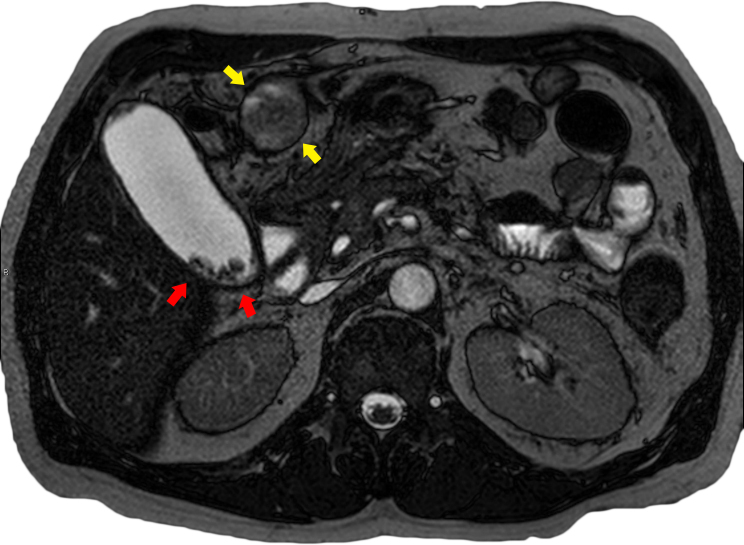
MRI *T*_2_ weighted transverse sequence again showing multiple small gallstones (red arrows). The paraumbilical vein is now significantly distended, measuring approximately 4 cm maximally (yellow arrows). Centrally, there is subtle high signal suggestive of slow flow with surrounding thrombus in varying stages of maturation and consequently of varying signal intensity. Surrounding the thrombosed vessel are significant inflammatory changes.
